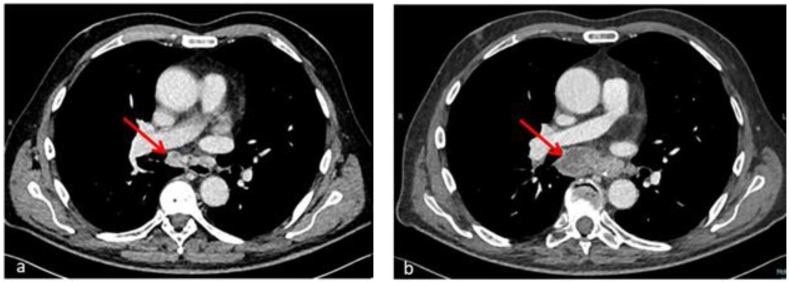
Axial chest CT sections after hematological recovery (b) compared with baseline examination (a) show enlargement of confluent hilar and mediastinal lymph nodes (66 × 26 mm vs. 24 × 15 mm) with necrosis and colliquation phenomena (red arrows) in an HCL patient with *Mycobacterium abscessus* infection. The radiological picture in the context of complete hematological remission is consistent with immune reconstitution syndrome.
